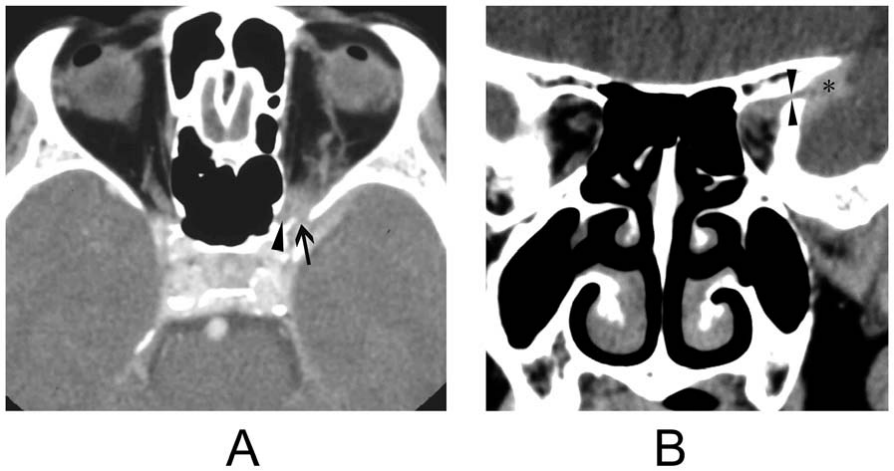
Idiopathic orbital inflammation syndrome with middle cranial fossa involvement. Figure 1A: Axial contrast-enhanced CT imaging reveals a lesion in the left orbital apex that extends through the superior orbital fissure (arrow) into ipsilateral middle cranial fossa. Note the involvement of the left optic canal (arrowhead). Figure 1B: Coronal contrast-enhanced CT imaging reveals a lesion in left orbital apex that extends through the superior orbital fissure (opposing arrowheads) into the ipsilateral middle cranial fossa (asterisk).
